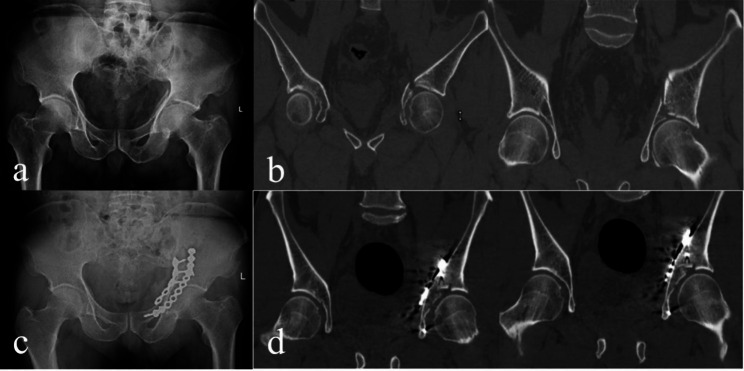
A male patient, aged 64, who presented with anterior column and posterior hemi-transverse fracture from a traffic accident. (a, b) Preoperative X-ray and CT scan revealed acetabular dome impaction fractures. (c) Postoperative X-ray showed satisfactory reduction of the fracture. (d) Postoperative CT showed 3.7 mm step displacement of the acetabular dome
